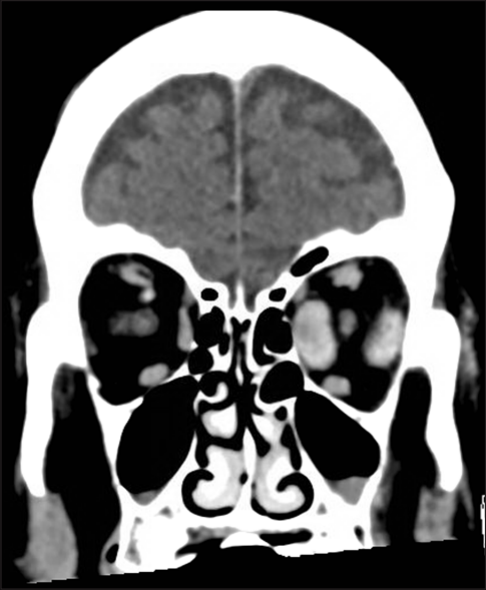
Coronal reconstructions of contrast-enhanced brain CT show fusiform thickening of the left medial and lateral rectus muscles as well as slight thickening of the left superior rectus muscle.
